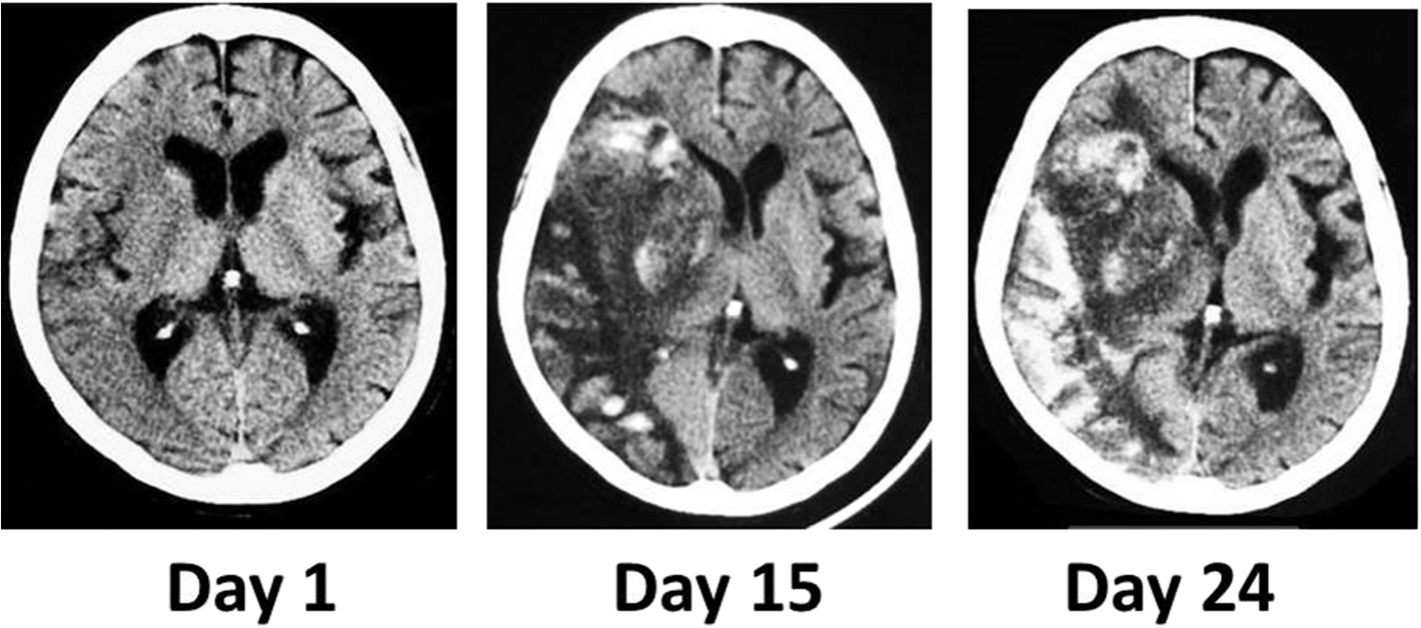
Changes of cerebral infarction in computed tomography. Slight, broad low density area in the right middle cerebral artery territory was depicted on day 1, showing the early cerebral infarction. Large low density area with midline shift and high density was depicted on day 15 and day 24, showing hemorrhagic transformation and severe brain edema.
